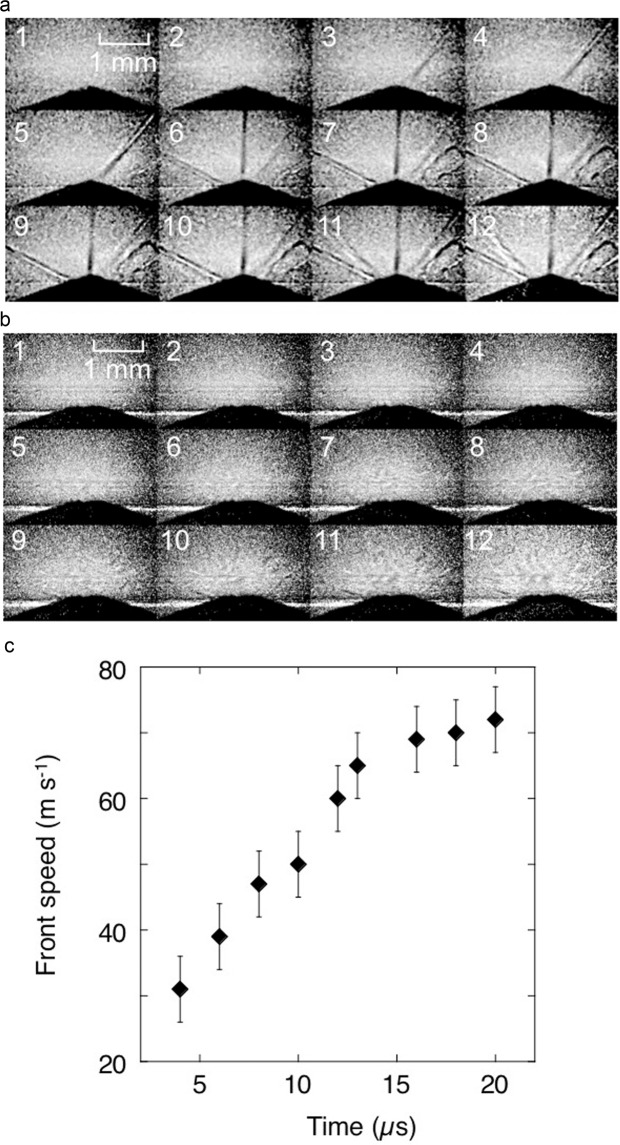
Impact of a triangular prismatic indenter onto (a) quartz (b) borosilicate targets. (c) Evolution of the damage region front velocity in borosilicate glass. X-ray image sequences have an inter-frame time of 1.404 μs.
